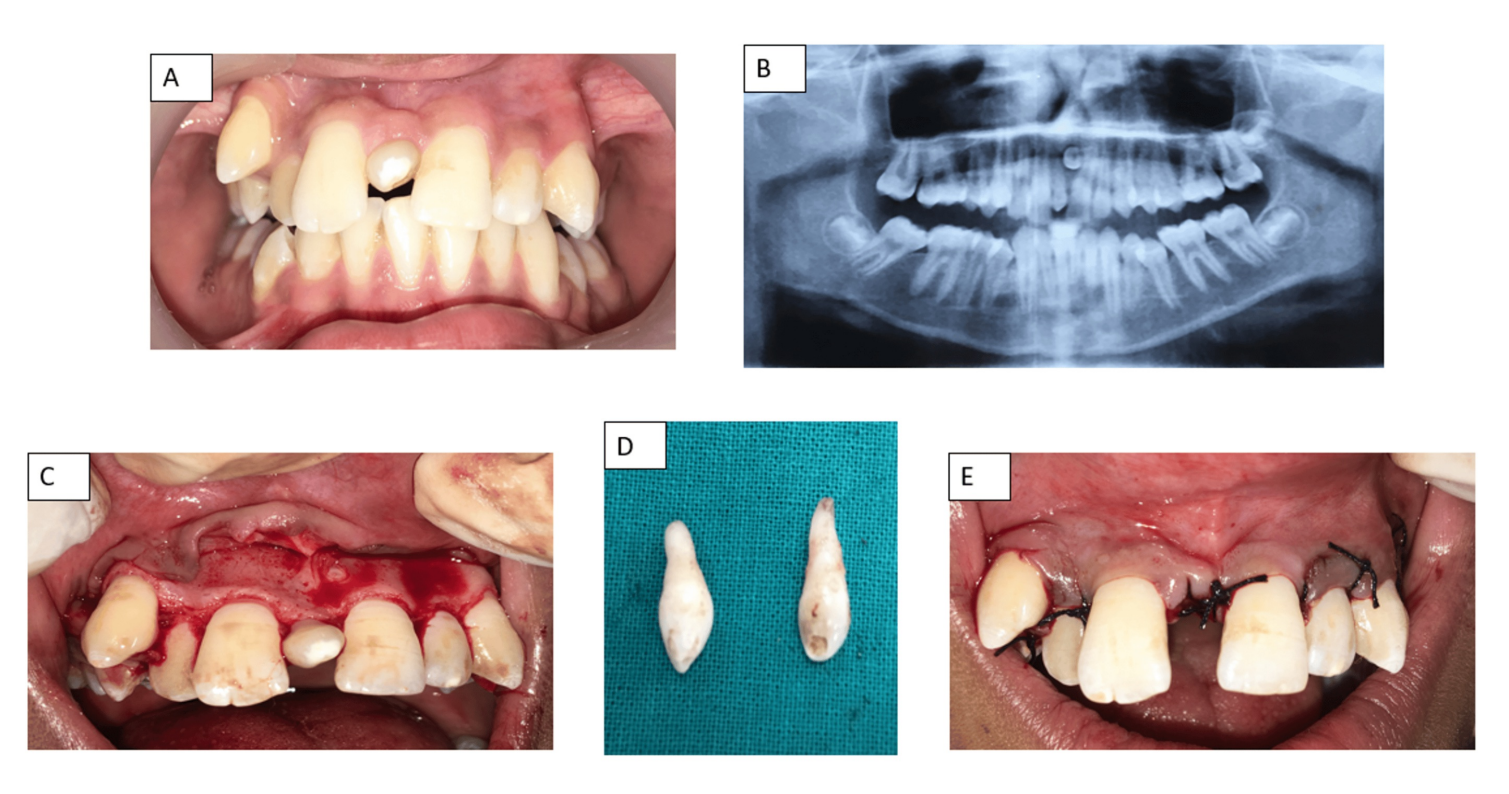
(A) Preoperative view of the maxillary anterior supernumerary tooth (conical type); (B) Orthopantomogram showing two supernumeraries; (C) Labial full-thickness mucoperiosteal flap raised; (D) Extracted two supernumerary teeth; (E) Postoperative suturing with 3-0 silk sutures
